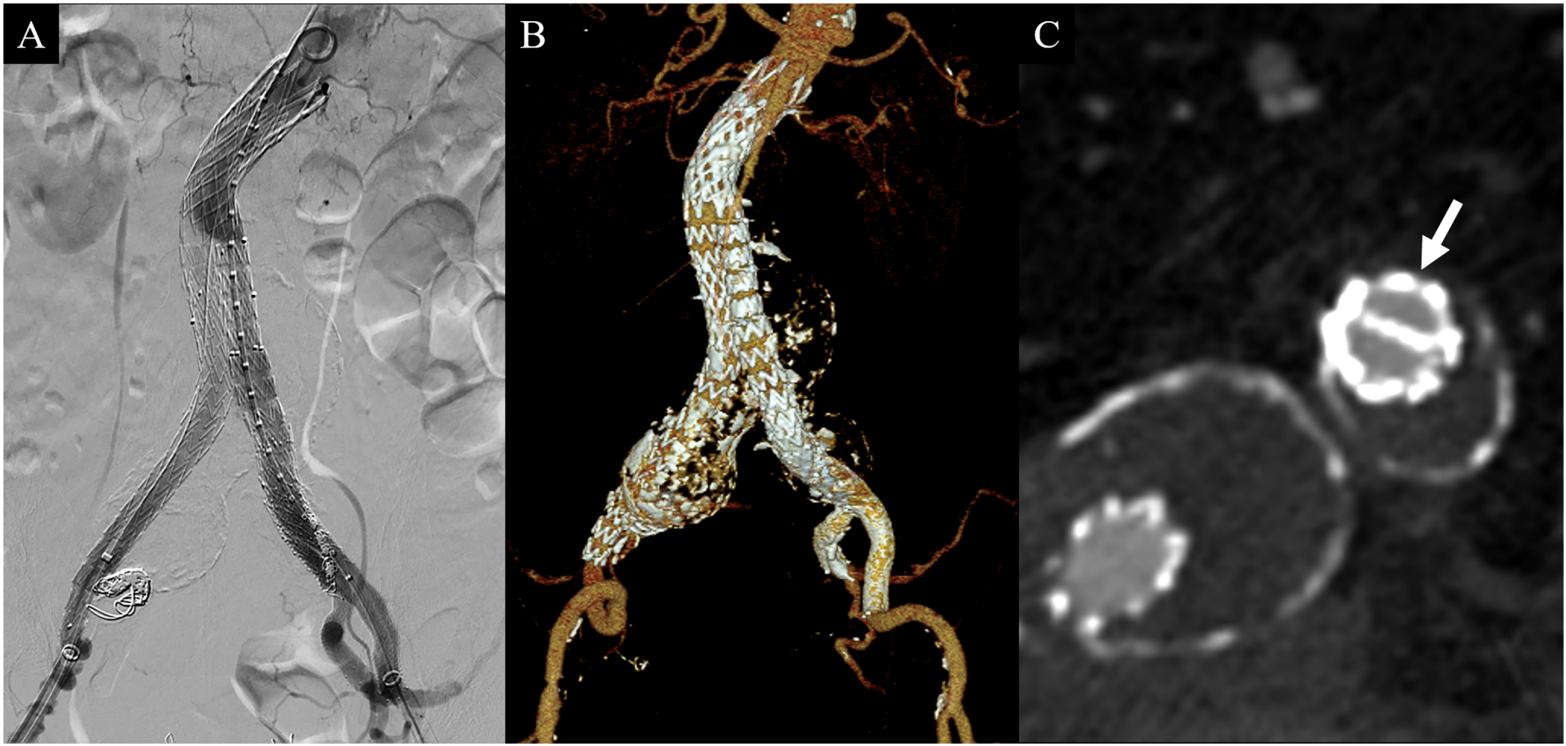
Final angiography during the operation and computed tomography angiography (CTA) 1-month postoperatively. (A) Final angiography after gutter embolization and Palmaz stent placement revealing no type Ia or gutter endoleaks. (B) 3-dimensional reconstruction image of follow-up CTA demonstrating well-patent parallel stent grafts without gutter endoleak. (C) Proximal portion of the parallel stent graft showing a double D configuration (arrow) without endoleak.
